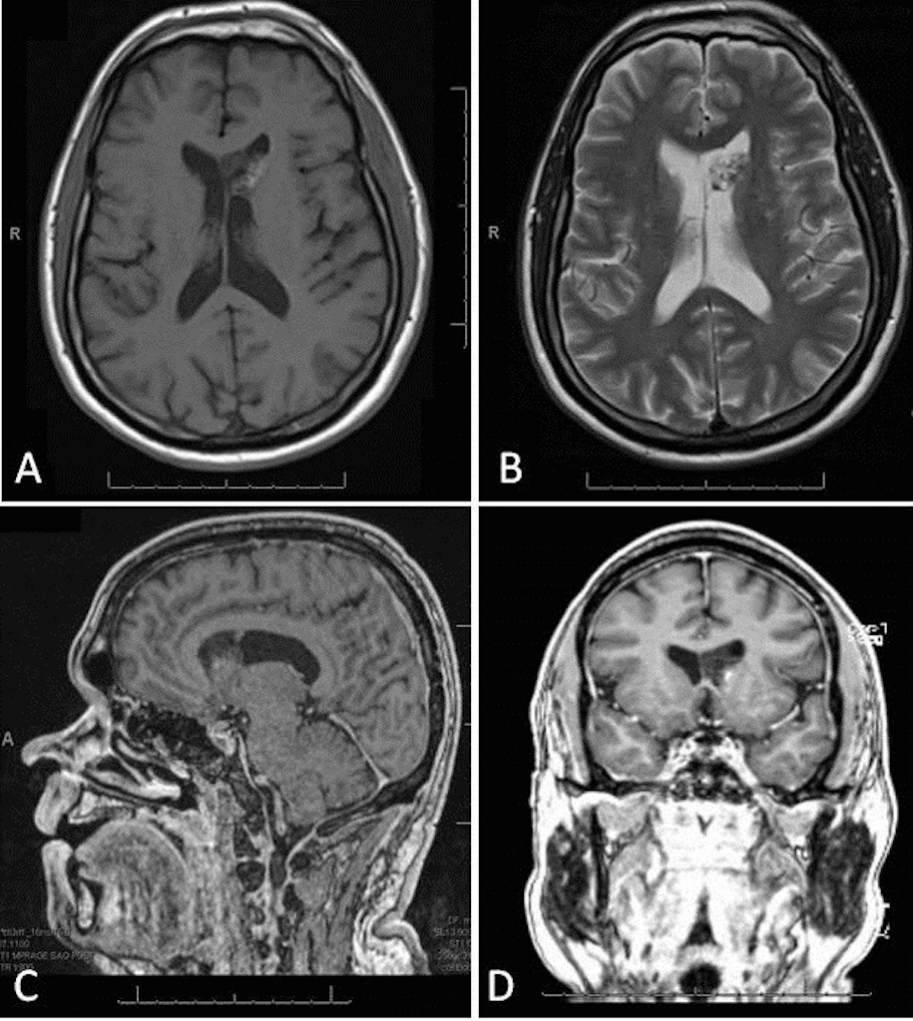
Preoperative MRI Brain Axial T1- (A) and T2- (B) weighted MRI demonstrating a cystic-like lesion within the left frontal horn of the lateral ventricle. Part of the lesion is hyperintense to grey matter on the T1-weighted images and hypointense on the T2 images. Sagittal (C) and coronal (D) gadolinium enhanced T1-weighted MRI showing no definite tumor enhancement.
